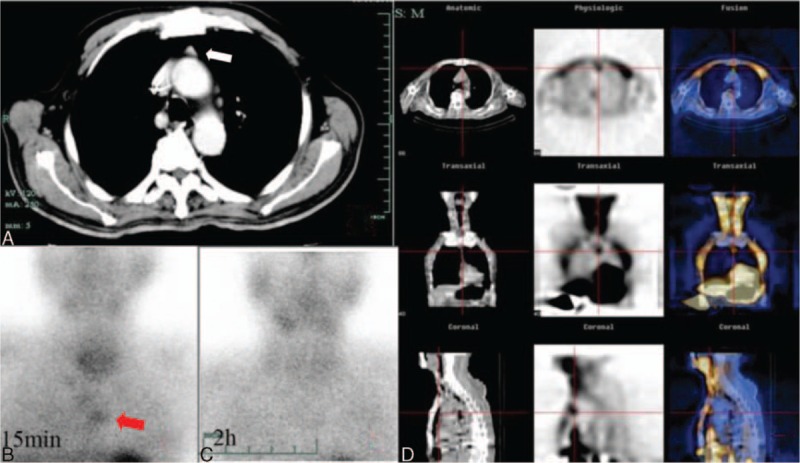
Contrast-enhanced CT of the thorax showed a well-defined soft tissue mass in front of the aortic arch in the upper-anterior mediastinum (A); 99mTc-MIBI showing early tracer uptake by thyroid glands and the gland in superior thorax at 15 minutes (B); rapid 99mTc-MIBI clearance in the superior mediastinum at 2 hours delayed phase (C); the accurate localization of mediastinal ectopic parathyroid adenoma with SPECT/CT (D). CT = computed tomography, MIBI = sestamibi, SPECT = single photon-emission computed tomography.
